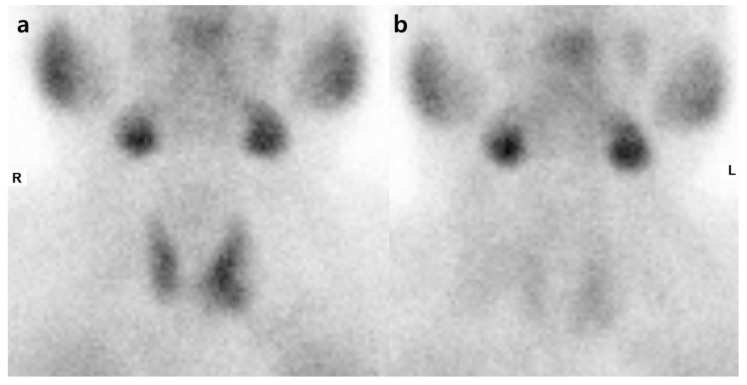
Dual-phase Tc-99m MIBI planar scintigraphy. The early images (a) show homogenous radioactive uptake in both lobes of the thyroid (enlarged left lobe) and the delayed images (b) show washout of radioactivity from the thyroid gland with no abnormal retention. R: right; L: left.
